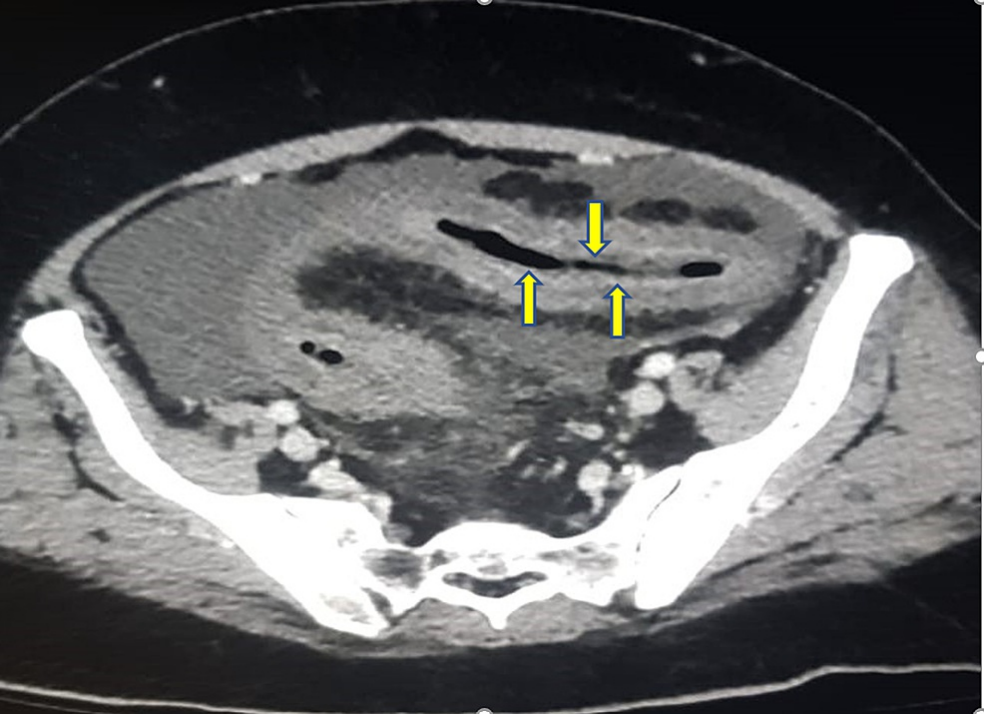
Computed tomography showing sigmoid colon mural thickening and luminal narrowing with decreased enhancement (yellow arrows).
